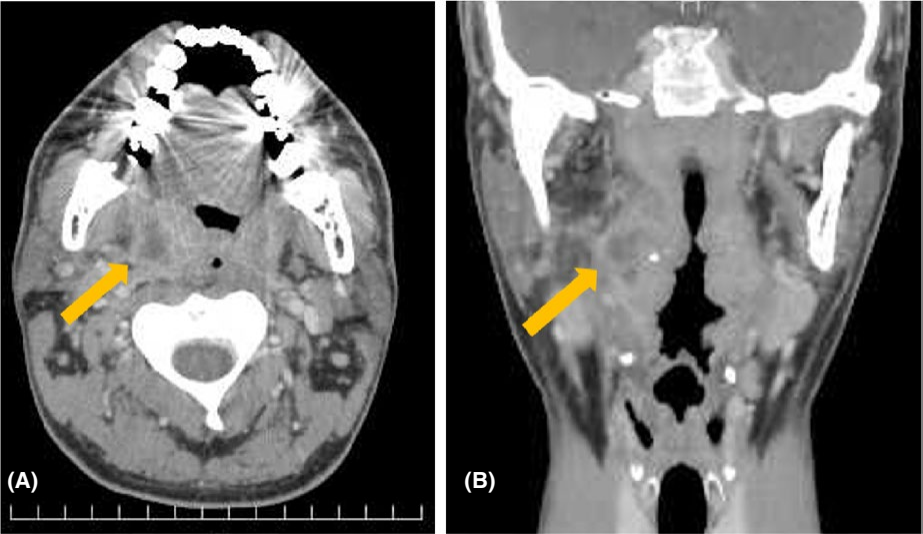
Contrast‐enhanced CT. Yellow arrow indicates peritonsillar abscess. A, Axial view; B, Coronal view
